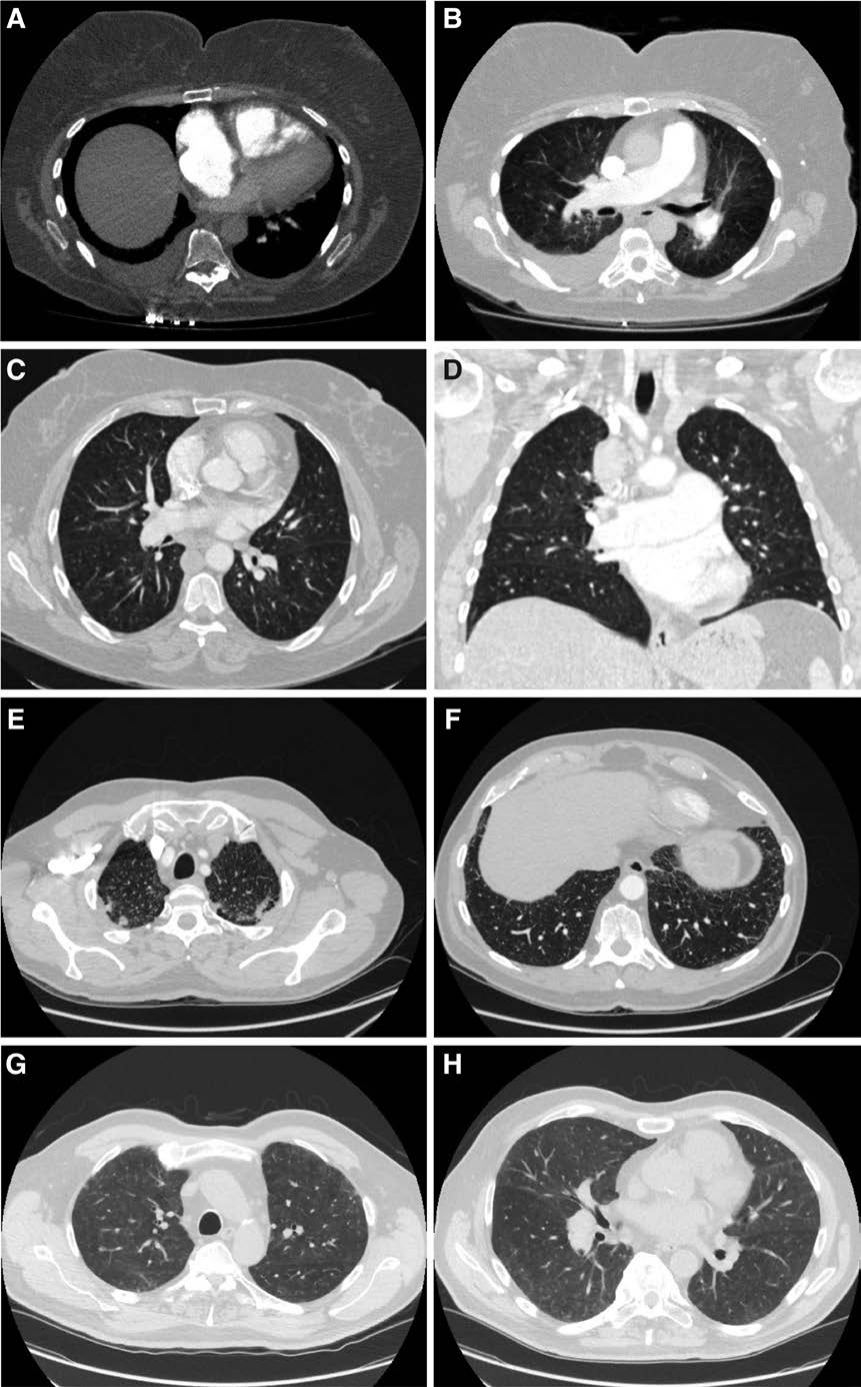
Chest computerized tomography (CT) scans of patients carrying high impact kinase insert domain receptor (*KDR*) mutations.
